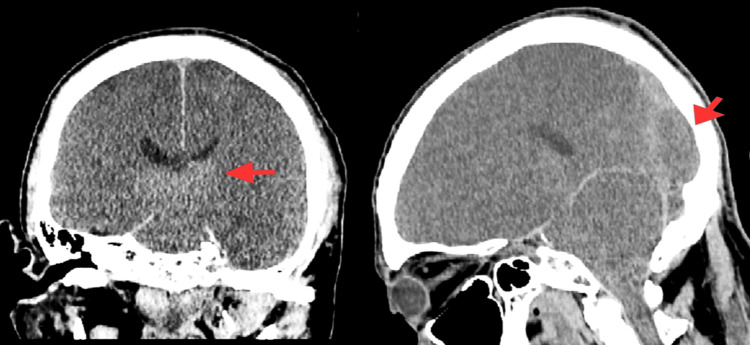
Follow-up non-contrast CT brain showing progression of diffuse cerebral edema, characterized by loss of gray-white differentiation, sulcal effacement, and ventricular compression Red arrows indicate areas of cerebral swelling.
